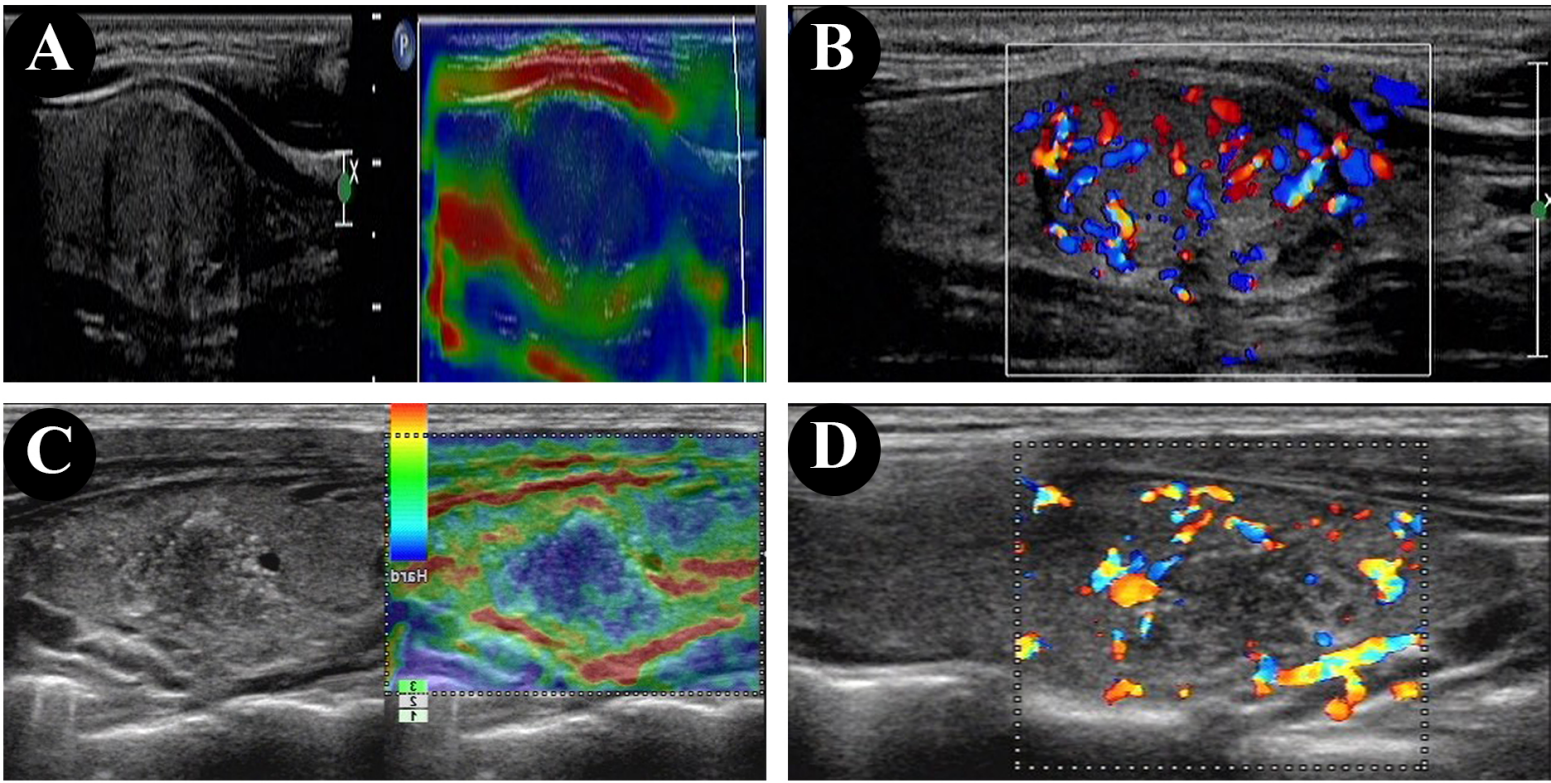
Ultrasonographic and elastography findings of PTC showed the tumor is closely attached to the capsule in the patient without HT (A, B), and extrathyroidal extension in the patient with HT (C, D).
